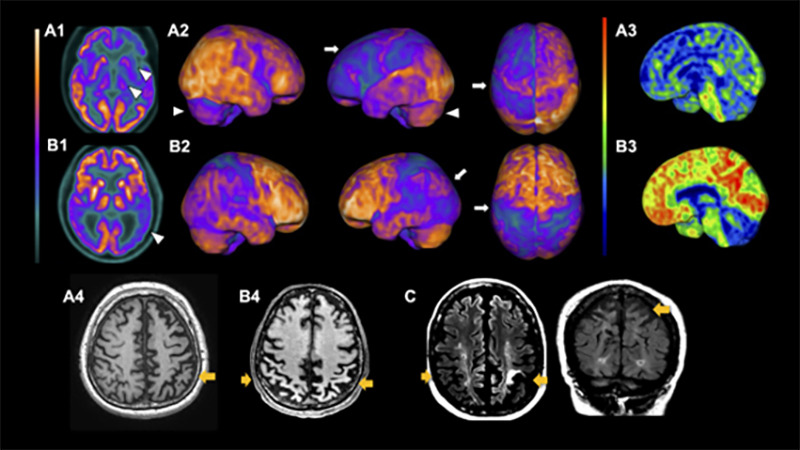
Neuroimaging features of CBD and CBS. Upper row: (A1) FDG-PET axial images of an individual with corticobasal degeneration (CBD) shows the classical asymmetric hypometabolism in the left basal ganglia and thalamus (white arrowheads). Contralateral cerebellar hypometabolism due to diaschisis is seen on FDG-PET 3D-stereotactic surface projection (arrowheads in A2)(3D-SSP, software cortex ID suite, GE Healthcare). (A2) A severe and extensive asymmetric frontoparietal cortical hypometabolism is seen, also in the sensory and motor cortex, worst on the left side, with severe asymmetric impairment of the frontal lobes. (A3) PIB-PET in the left medial projection of the same patient tested negative for amyloid deposition. This pattern is consistent with CBS due to 4R tauopathy (CBD). Middle row: B1) FDG-PET axial images of an individual with corticobasal syndrome due to Alzheimer's Disease (CBS-AD). A posterior bilateral temporoparietal hypometabolism is seen, only slightly asymmetric, without impairment of the basal ganglia, thalami, and cerebellum (white arrowheads). B2) FDG-PET 3D-stereotactic surface projection shows a severe and extensive hypometabolism predominantly in the parietal lobes, however also including the sensory and motor cortex, slightly worse in the left side, with the left side relative sparring of the frontal lobes. (B3) PIB-PET in the left medial projection shows diffuse cortical amyloid deposition. This is the typical pattern related to CBS-AD. Lower rows - atrophy patterns seen on CBS. (A4) T1-weighted MRI with asymmetric frontoparietal atrophy, also worst on the left side (orange arrow) of the same patient on A1,2 and 3. (B4) MRI of the same patient with CBS-AD shown on B1,2 and 3, showing bilateral parietal cortical atrophy (orange arrows). (C) T2/FLAIR MRI shows severe atrophy and asymmetric subcortical parietal gliosis, especially of the perirolandic cortex on the left side, on a patient with late-stage CBD.
